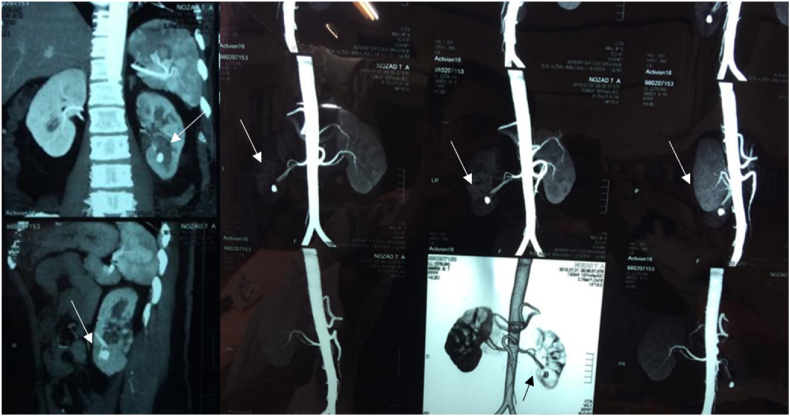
The arrows show pseudoaneurysm in the lower calyx of kidney in different views of CT angiography.
